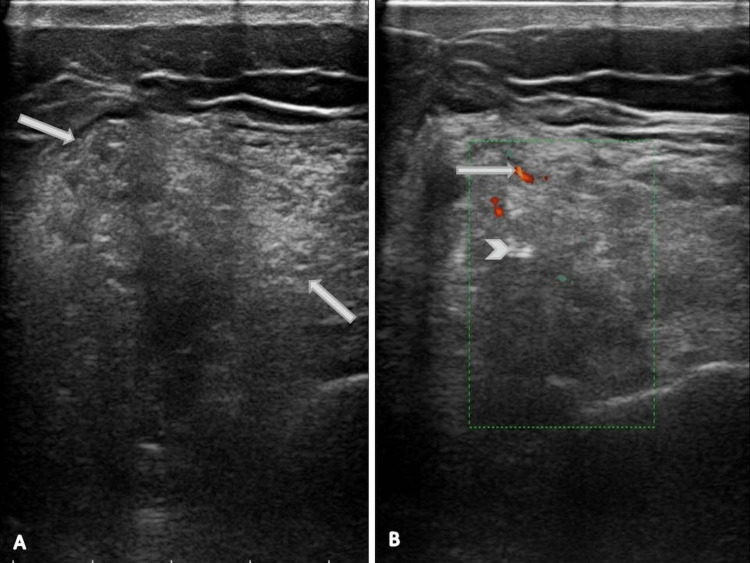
Ultrasound shows (A) a well-defined, spindle-shaped, predominantly echogenic lesion without compressible venous channels. (B) Colour doppler shows minimal venous flow. The lesion shows the presence of calcification
